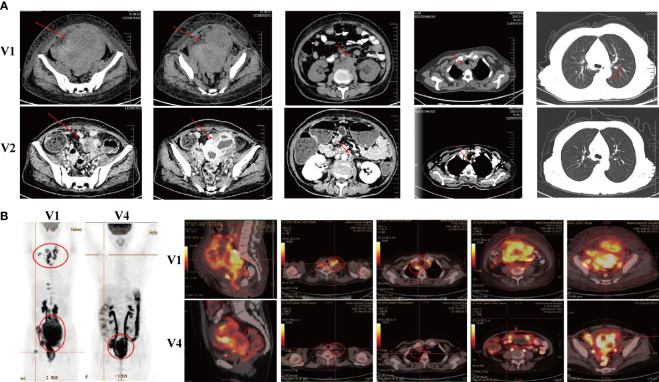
Radiological evaluations of the patient at different time points. (A) CT scans were conducted before (V1) and approximately two months (V2) after the initial treatment course. (B) PET-CT scans were performed before (V1) and approximately eight months (V4) after the initial treatment course.
